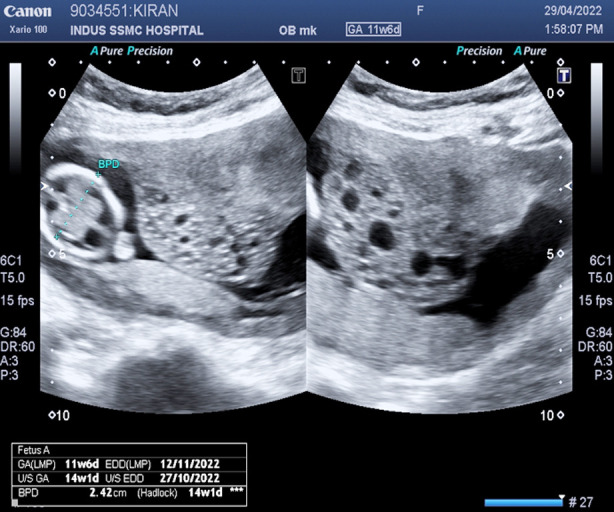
Ultrasound at 11+6 weeks showing fetus with normal placenta and complete hydatidiform mole.
